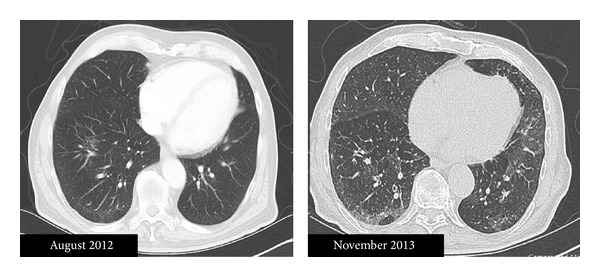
Computed tomography image of the patient's lungs prior to treatment with chlorambucil (August 2012) and after diagnosis of pneumonitis (high-resolution scan, November 2013), demonstrating interval development of interlobular septal thickening more prominent in the periphery of the right lung, suggestive of interstitial lung disease.
